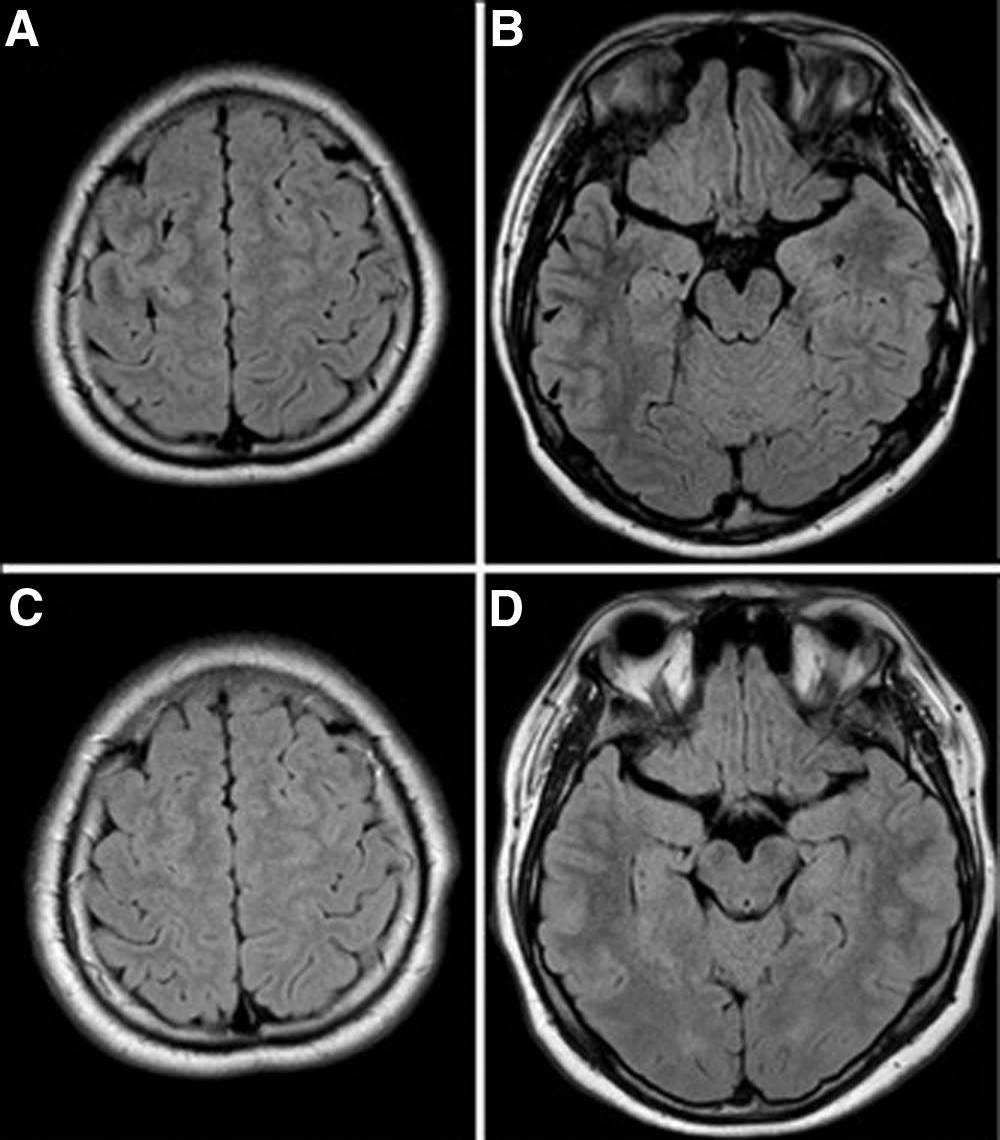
Brain MRI findings of the patient. (A and B) Brain MRI revealed slightly high signal intensity in the right frontal cortex, low signal on T2/FLAIR in right frontal subcortical white matter, and high signal on T2/FLAIR in the right temporal lobe. (C and D) Repeated FLAIR image showed that the low signal in the right frontal subcortical white matter had resolved, and the high signal in the temporal had disappeared after 6 months of treatment.
